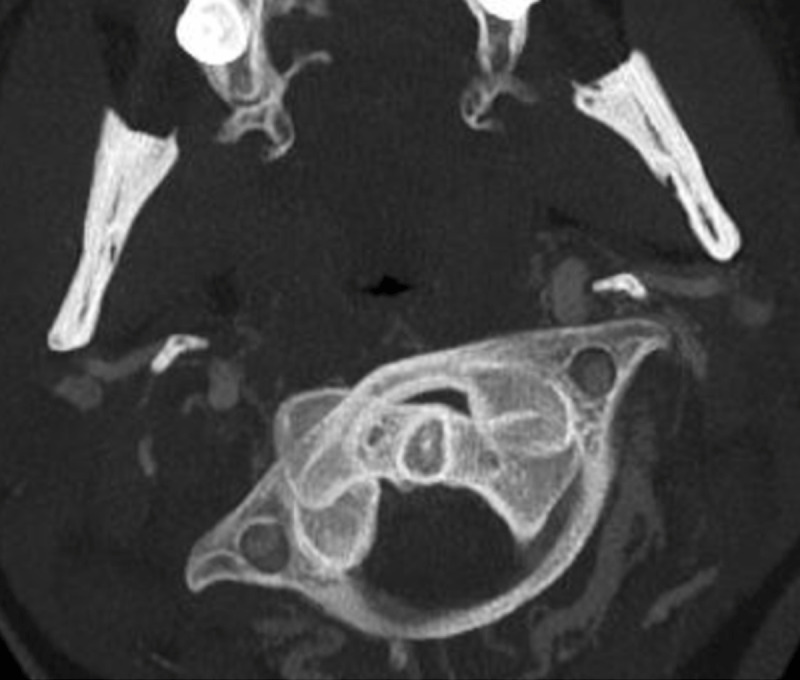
Rotation of C1 on C2 on CT in neutral position.
